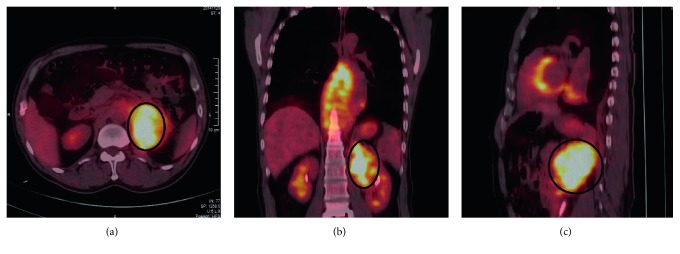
^18^F-FDG-PET/CT images of a 63-year-old male patient with non-Hodgkin's lymphoma on the left adrenal gland. He was alive at the end of our follow-ups with no disease progression. The green lines represent the borders of the VOI. The GLRLM_RLNU value of his PET images was 55.8 (lower than our cutoff value 216.6)
